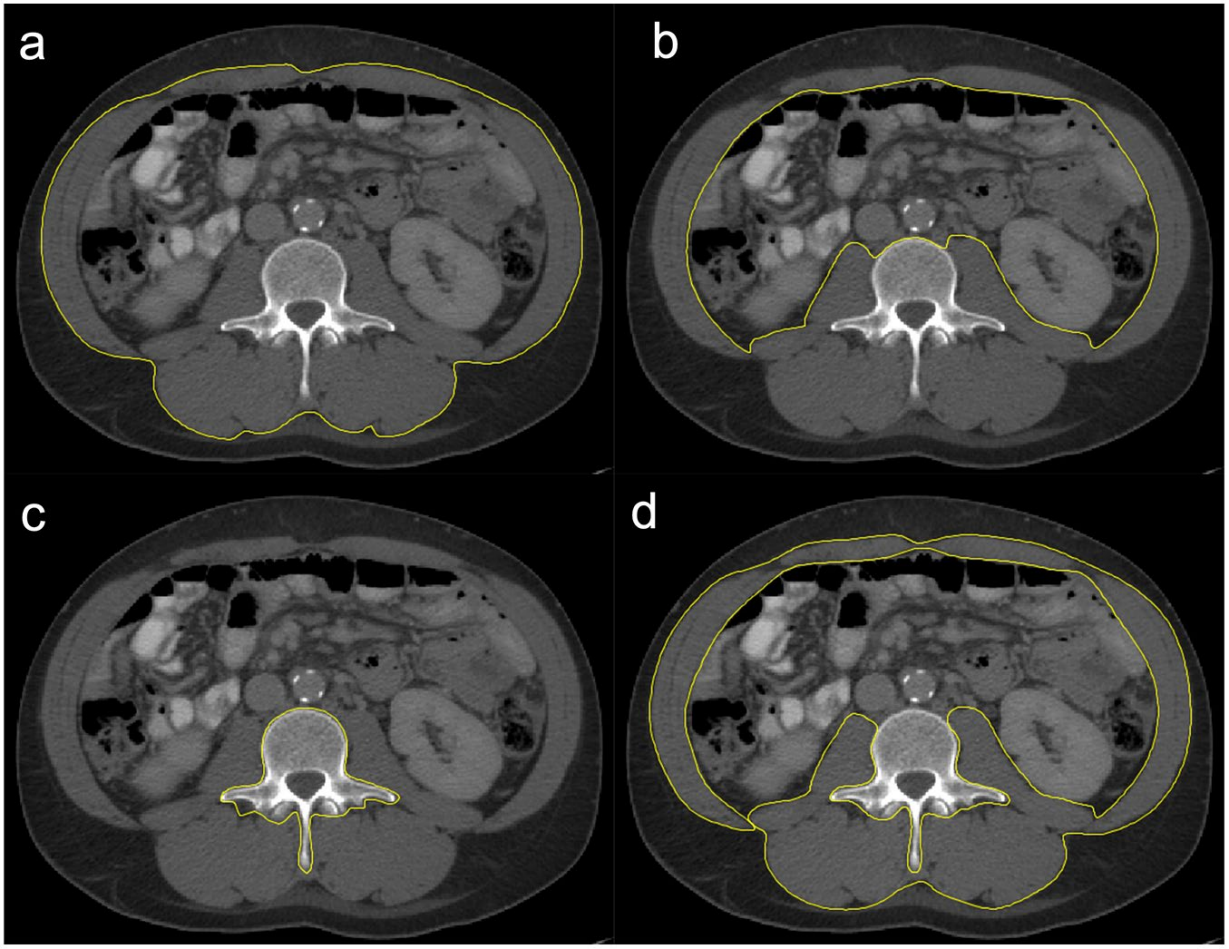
Regions of interest for CT muscle mass and muscle density assessment. Exemplary definition of regions of interest (ROIs) in a patient with high muscle status. ROIs were drawn (a) along the outer circumference of the whole abdominal muscle at the level of the third lumbar vertebral body (L3), (b) along the inner circumference of the abdominal muscle and L3, and (c) around L3. The skeletal muscle index (SMI) was calculated by: area of (ROI 1– ROI 2– ROI 3 [cm^2^])/(body height [m])^2^ after application of a muscle specific threshold of − 29 to + 150 Hounsfield units (HU). The muscle radiodensity attenuation in HU-values was derived from a ROI around the whole abdominal muscle area (d) after application of the muscle specific threshold.
